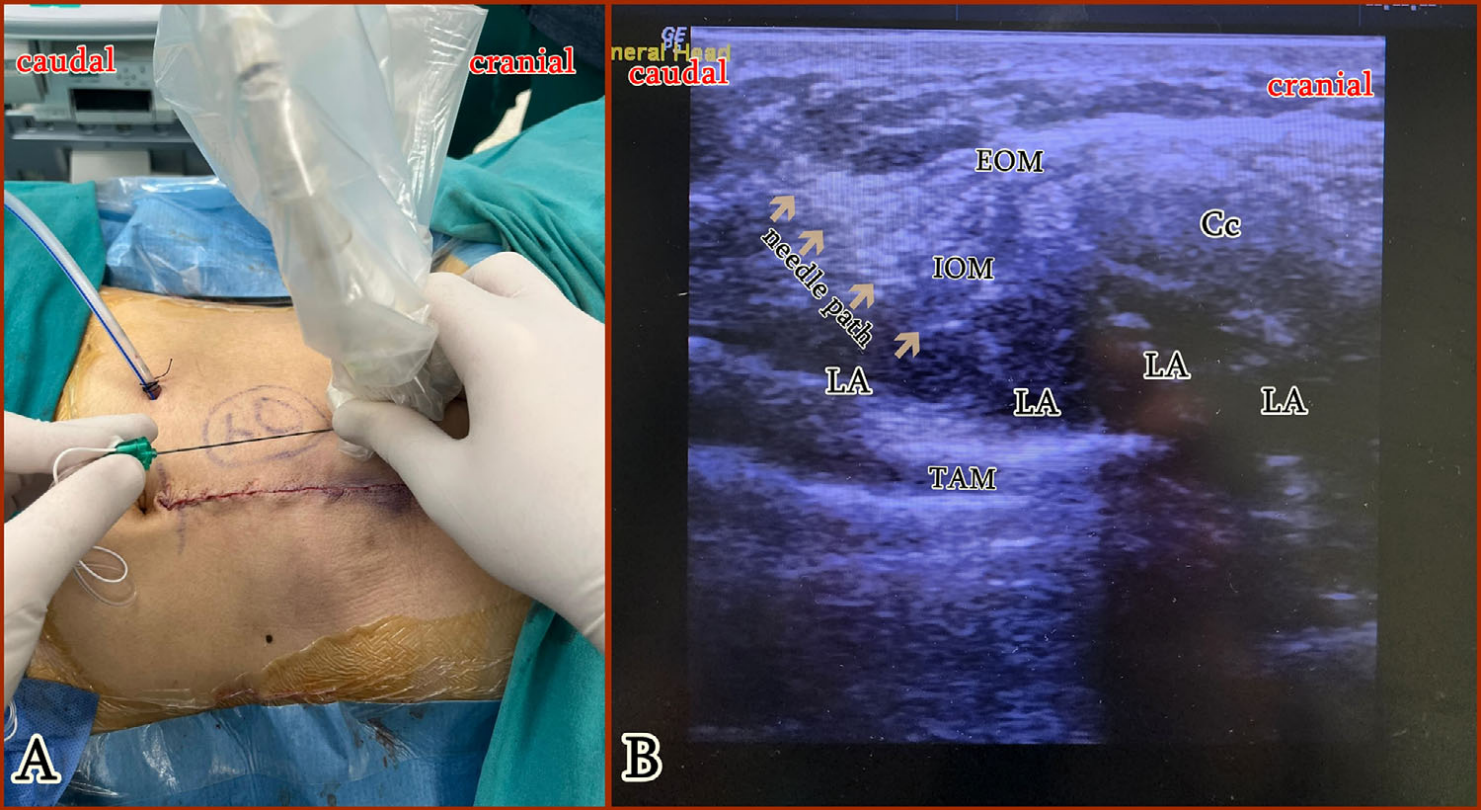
A. Patient and probe position during M‐TAPA block. B. Sonographic visualization of M‐TAPA block. 10th costal cartilage and spread of local anesthetic are seen. The arrows indicate the needle path. CC: costal cartilage, EOM: external oblique muscle, IOM: internal oblique muscle, LA: local anesthetic, P: perichondrium, TAM: transversus abdominis muscle.
